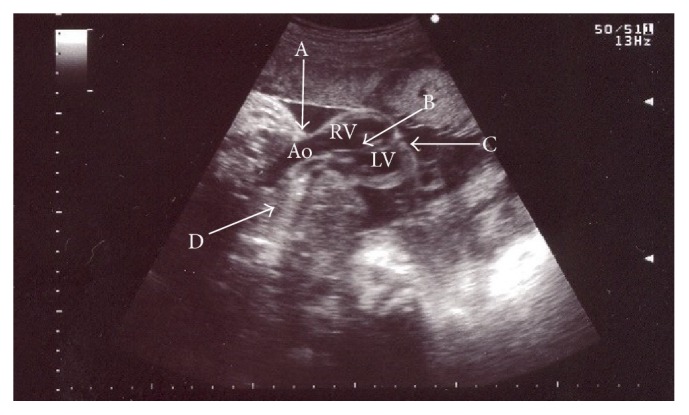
Ultrasound of fetus heart at 42nd week of gestation, thoracic cleft, and major blood vessels transposition. Ao: aorta, RV: right ventricle, and LV: left ventricle. A: wide anterior thoracic defect, B: ventricular septum, C: ectopic heart, and D: chest.
